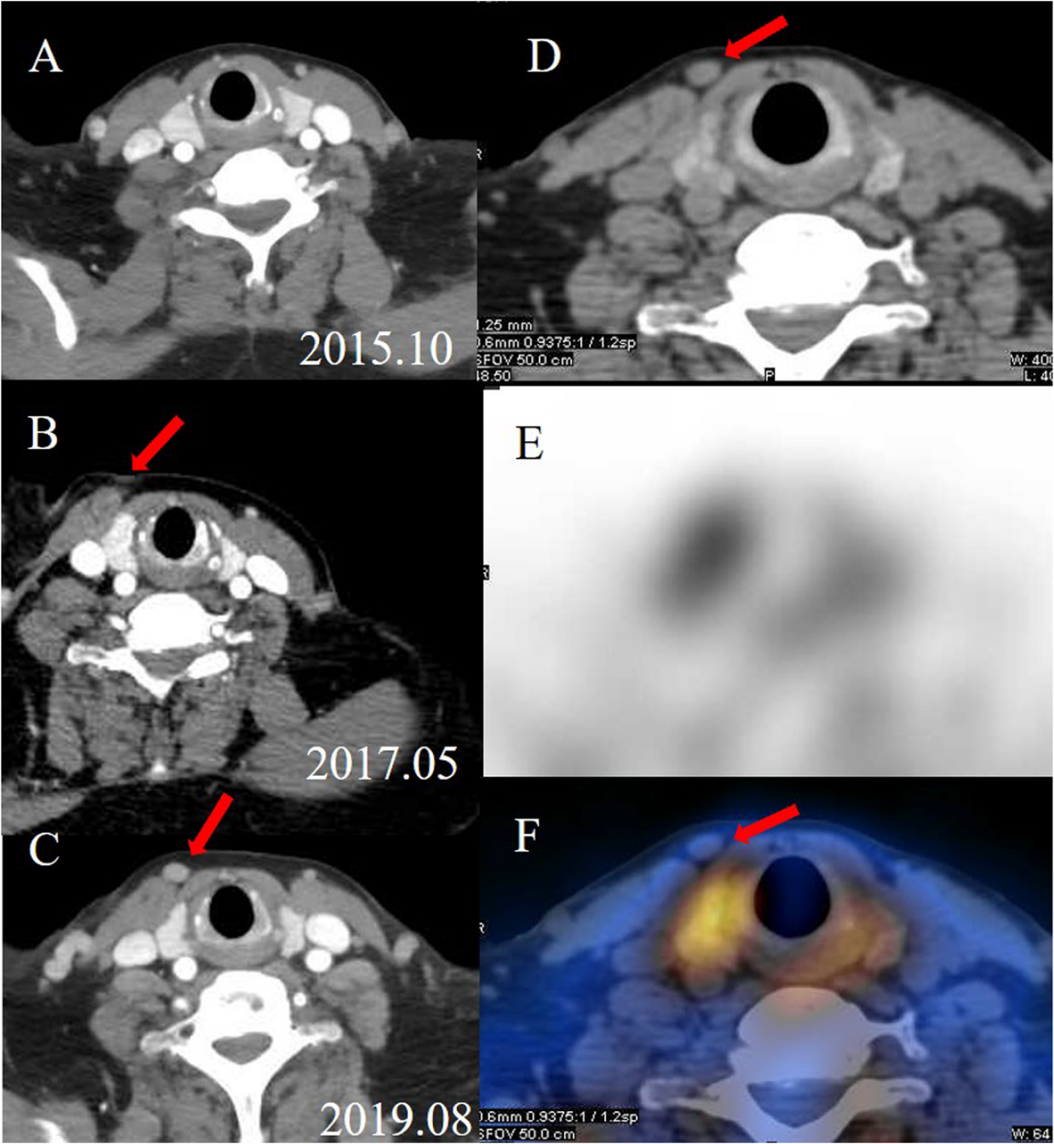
Serial arterial-phase axial 4D-CT of the parathyroid shows the growth of the right nodule (red arrows) located subcutaneously in the anterior sternocleidomastoid muscle (A, pre-operative; B, 18 months after surgery; C, 46 months after surgery). The ^99m^Tc-MIBI SPECT/CT image reveals the lesion without tracer uptake (red arrows: D, axial image; E, SPECT; F, fusion image)
